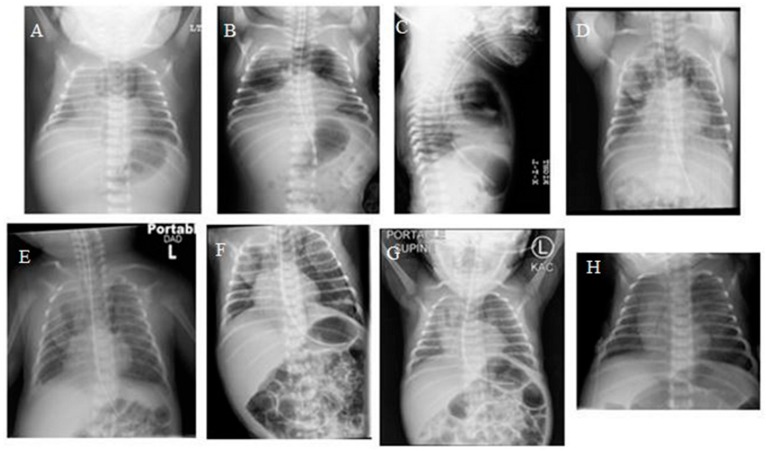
Serial plain chest AP radiograph showing progression of pneumomediastinum (PM) (A,B,D) and resolution (H); Lateral chest radiograph showing PM (C).
